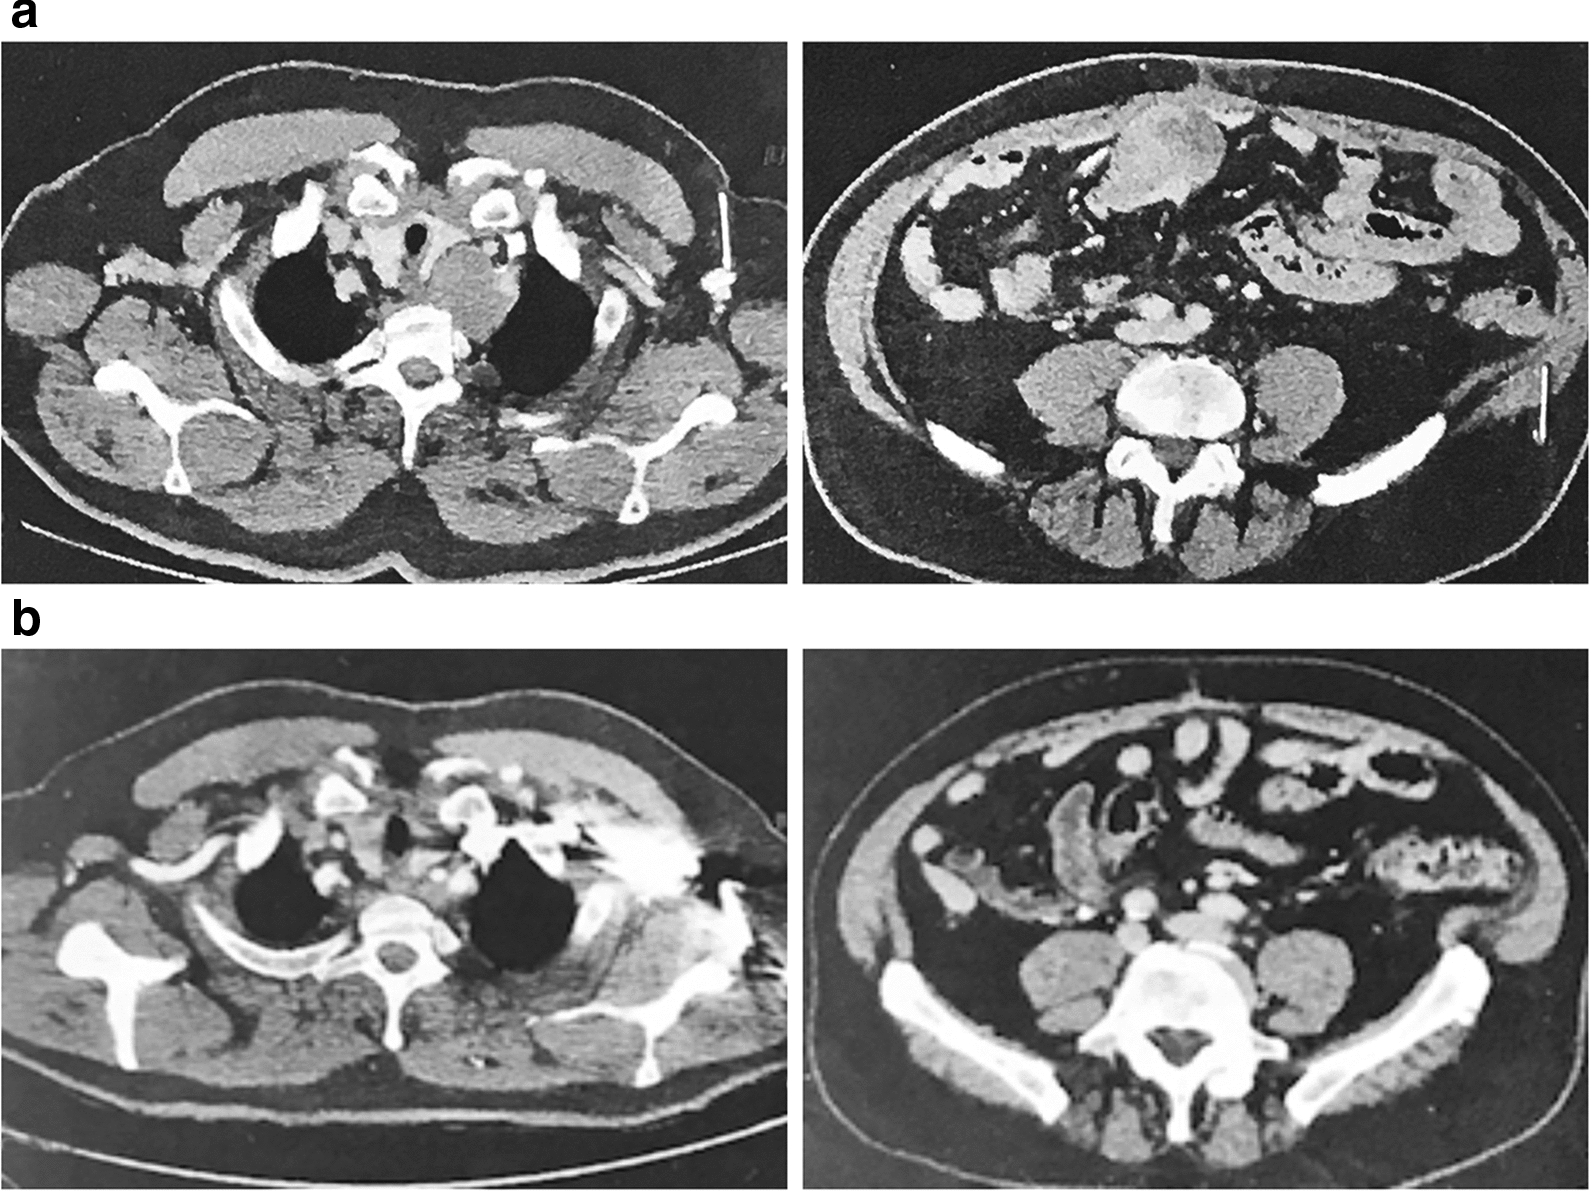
Computed tomography scans from a patient with metastatic Merkel cell carcinoma treated with avelumab. a Baseline and b complete response at 2 months after starting avelumab treatment. Images were provided by Dr. Corsi
